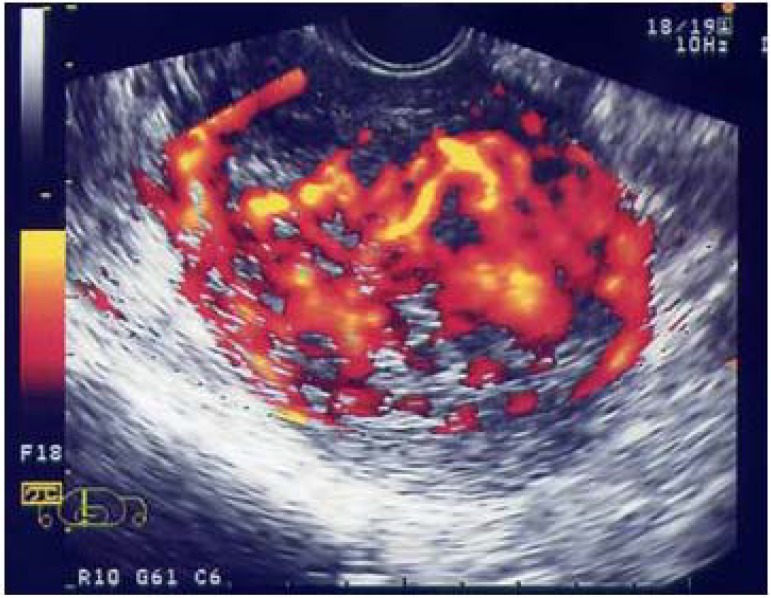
Potential blood flow (angiogenesis) of malignant tumors is enhanced and extracted using color Doppler ultrasound imaging. This picture is a clinical case of uterine sarcoma, using Levovist. Because uterine sarcomas have the most aggressive angiogenesis among all uterine cancers, they are used as a model for angiogenesis.
